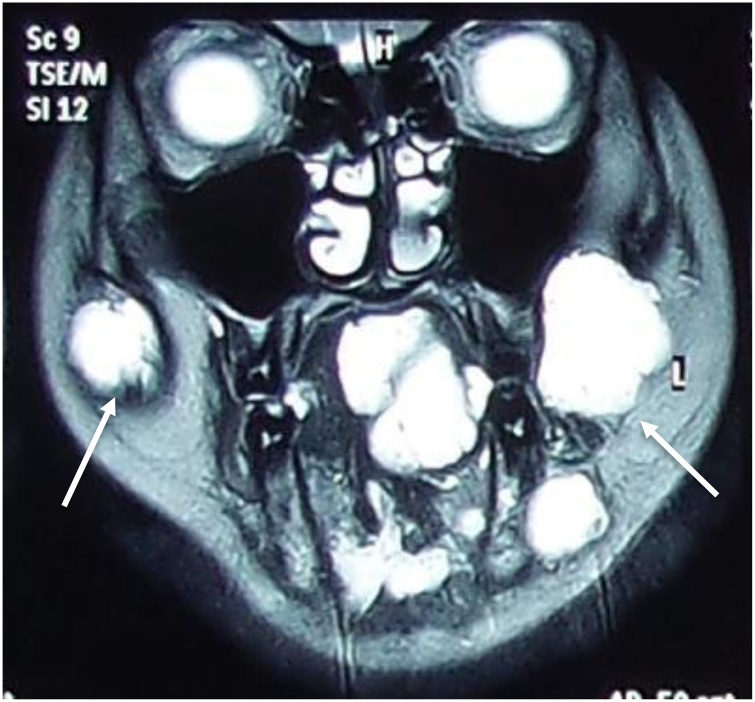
MRI above is showing multiple lobulated lesions over bilateral cheek soft tissue and tongue (white arrow).
